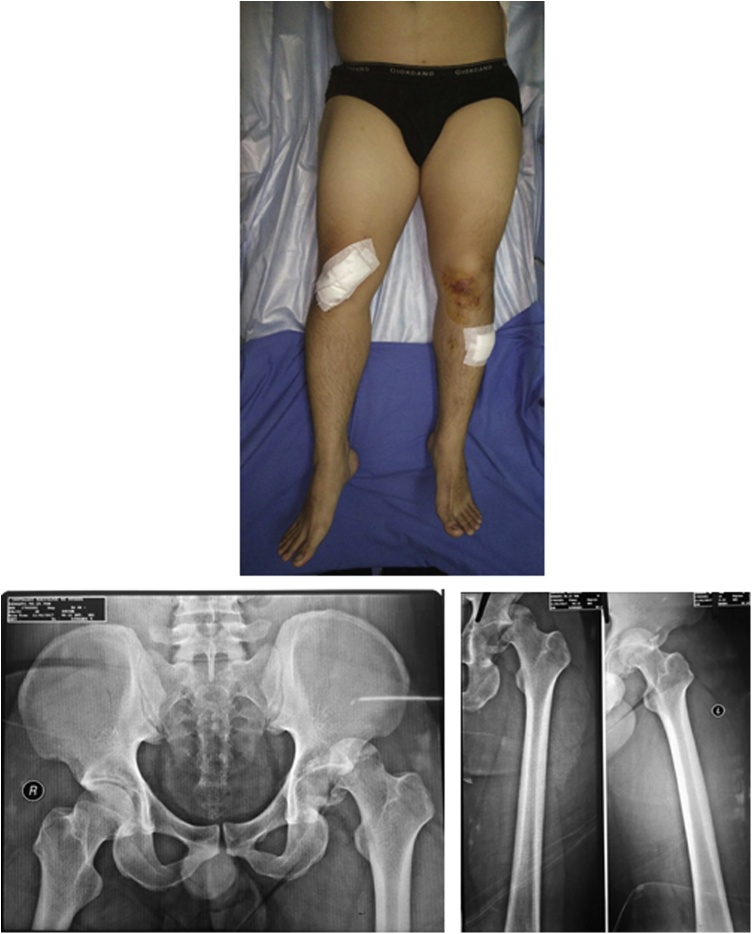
Local State of the Pelvic & Pelvic and Femoral X-Ray in The Emergency Room.
